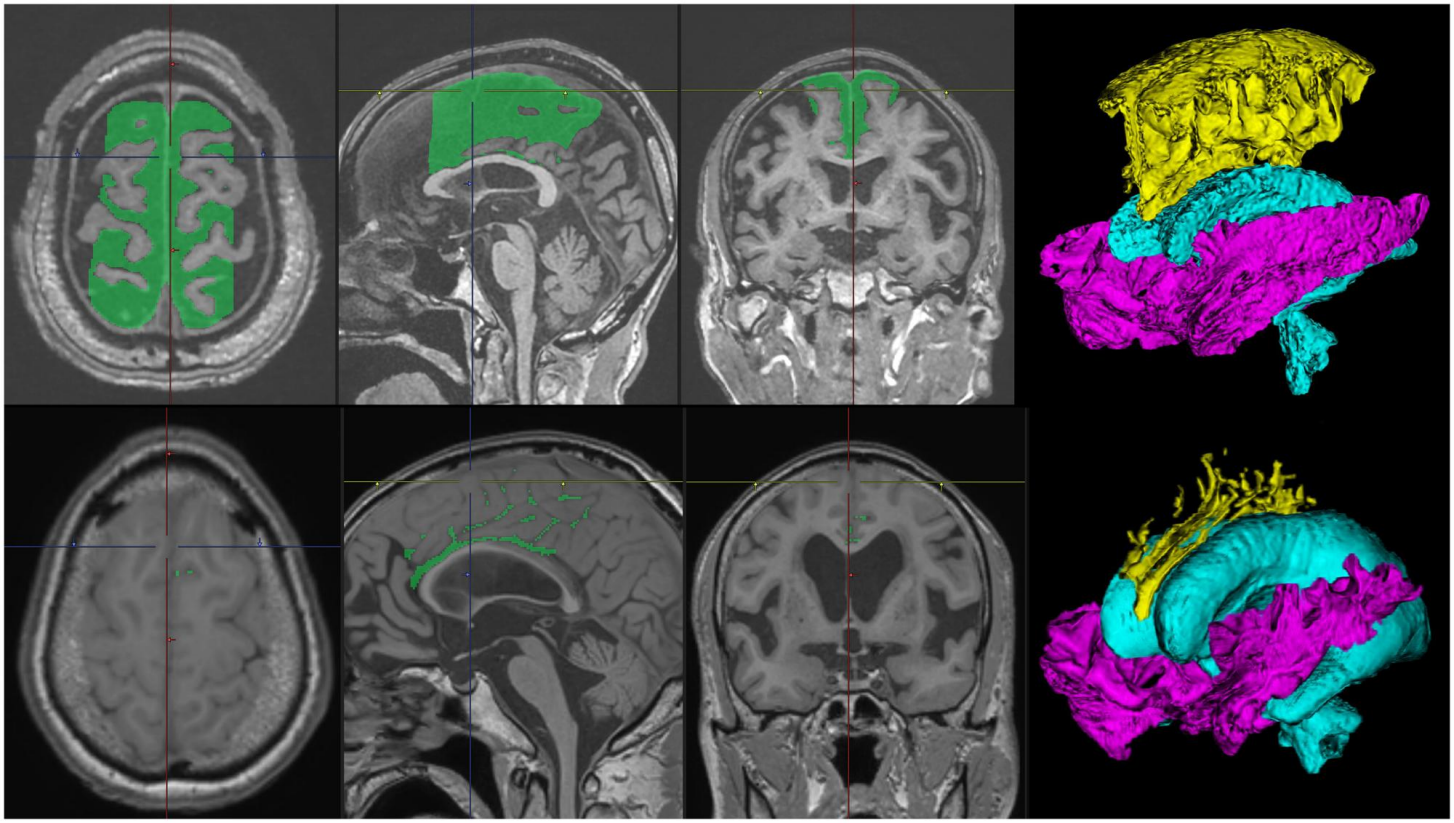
Segmentation of disproportionately enlarged subarachnoid space hydrocephalus (DESH) from 3D T1-weighted MRI in Hakim’s disease (idiopathic normal pressure hydrocephalus, iNPH). The cerebrospinal fluid (CSF) Space Analysis application on the 3D volume analyzer SYNAPSE 3D workstation (FUJIFILM Corporation) quickly and automatically segmented the 3 following regions of interest: the total ventricles (sky blue), high-convexity part of the subarachnoid space, and Sylvian fissure and basal cistern in the right figures. The highlighted regions in the left triaxial sectional views indicate the high-convexity part of the subarachnoid space. The upper figures show the brain of an elderly healthy volunteer with brain atrophy, whereas the lower figures show the brain of a patient with Hakim’s disease (iNPH) with a typical DESH feature.
